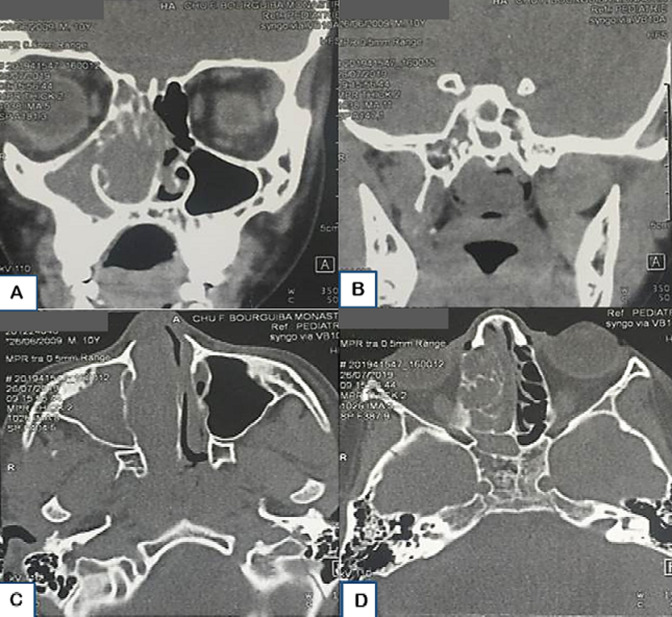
coronal (A, B) and axial (C, D) CT scan showing a large mass of the right nasal cavity with an extension to the choana (A, C) and nasopharynx (B) with a right ethmoidal filling (D)
